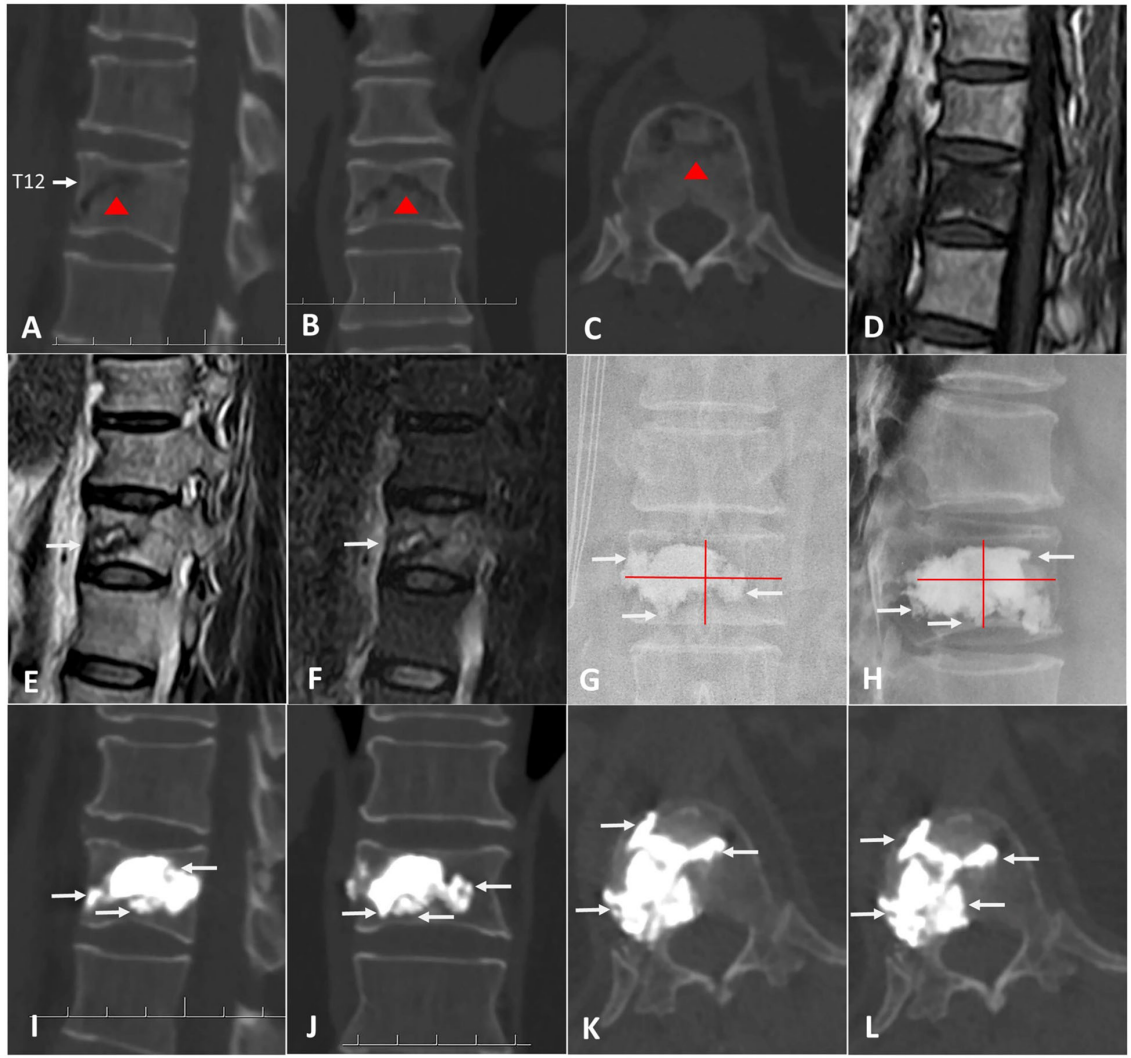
A 74-year-old man with KD at T12 was treated by A-PKP. (A-C) Preoperative CT showing IVC (red triangle). (D) the sagittal T1-weighted MRI showing low signal intensity in IVC. (E, F) the sagittal T2-weighted and STIR sequences MRI showing high signal with surrounding low intensity (Double-line sign; DLS). (G, H) postoperative X-ray exhibiting spongy cement pattern. (I-L) CT scans at final follow-up showing satisfactory cement anchorage (white arrows).
